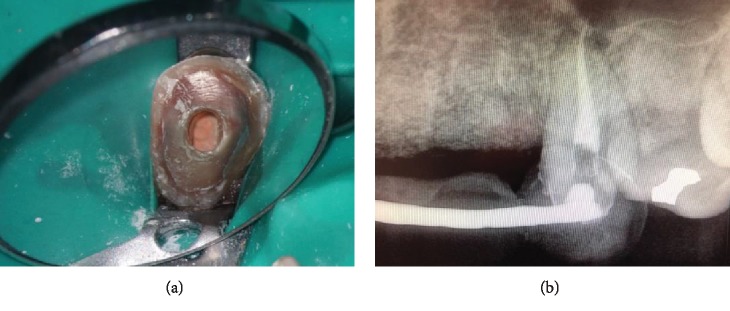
(a) Clinical photograph of the obturated tooth #11 prior to placing a temporary filling and the provisional FPD. (b) Postoperative radiograph of the obturated tooth #11 with the provisional FPD cemented.
